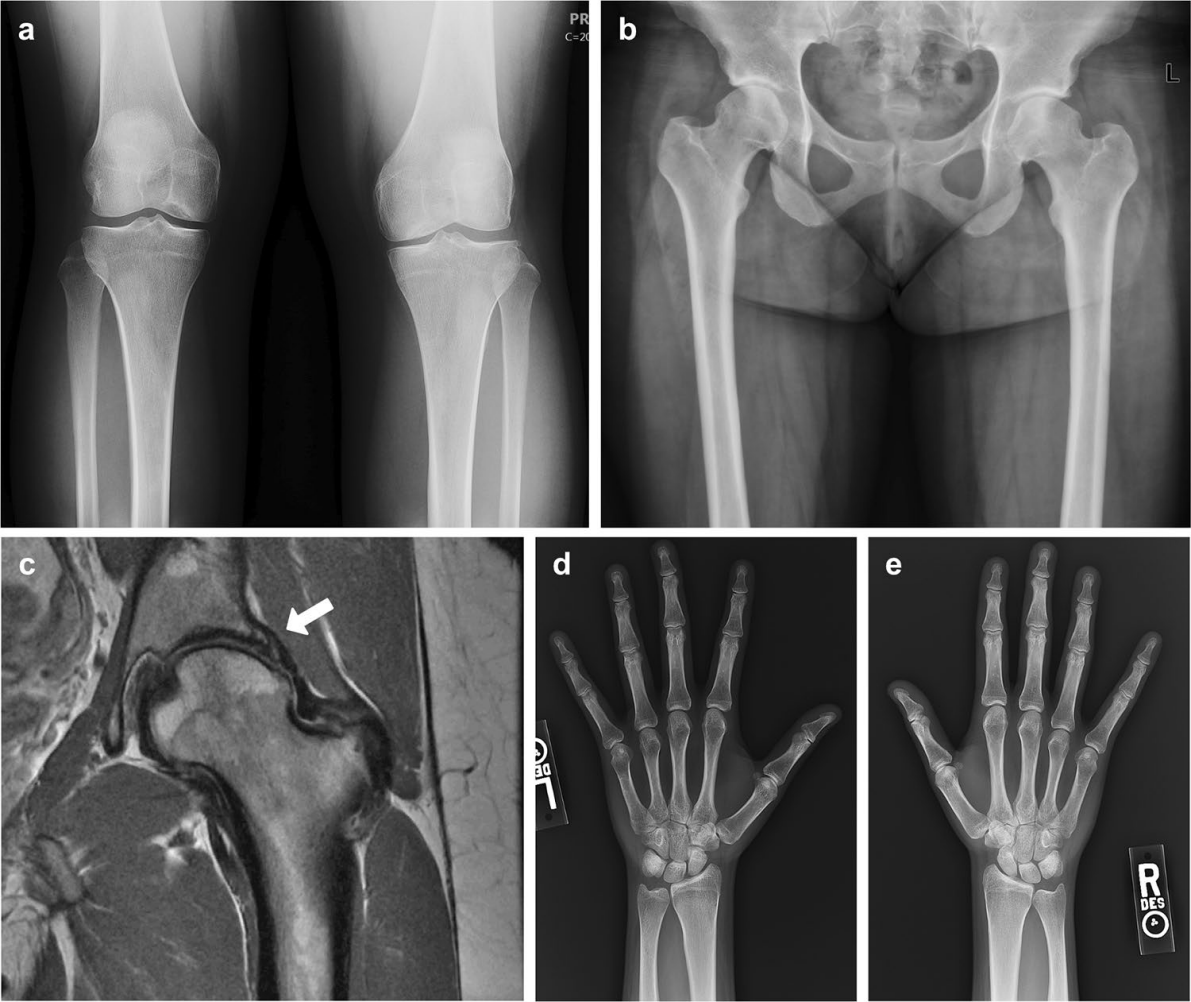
Clinical observations in Family 3. The proband is a now 33-year-old woman, 156 cm tall, with joint pain, early onset osteoarthritis and osteochondral lesions in multiple joints, including knees (a) and hips (b). She has had hip arthroscopic treatment for femoral-acetabular impingements and labrum tears (c, arrow). Hand X-rays show short and broad distal phalanges of the thumbs (d,e). She has a family history of short stature and joint problems on the paternal side, including OCD.
